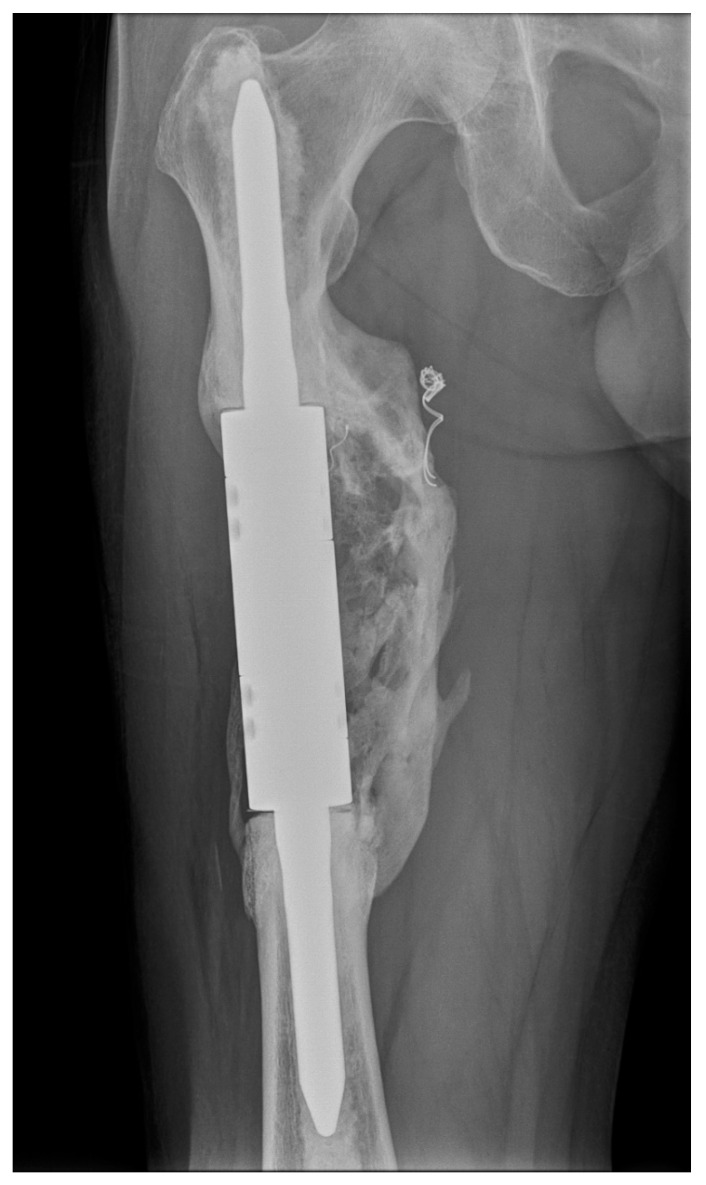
X-ray image of an intercalary endoprosthesis with the formation of heterotopic ossification around the implant after the resection of a renal cell carcinoma metastasis, with a bone bridge connecting the proximal and distal bone fragments. This image is from patient 1 in Table 1.
